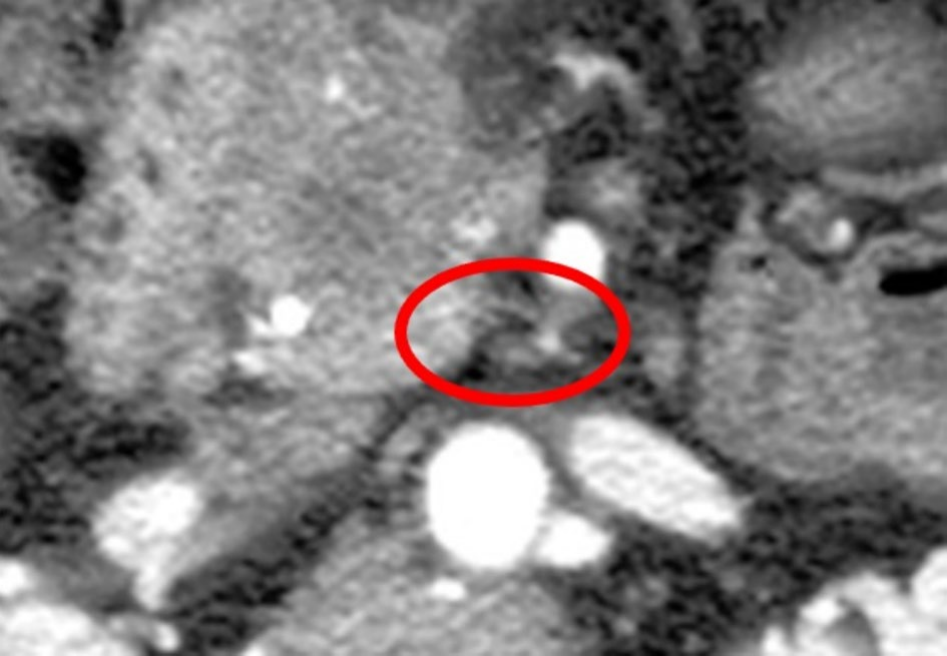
Axial CT scan in arterial phase shows Pattern 2 of EPNI, that represents infiltration along the IPDA in the reticular pattern without tumor contact.
